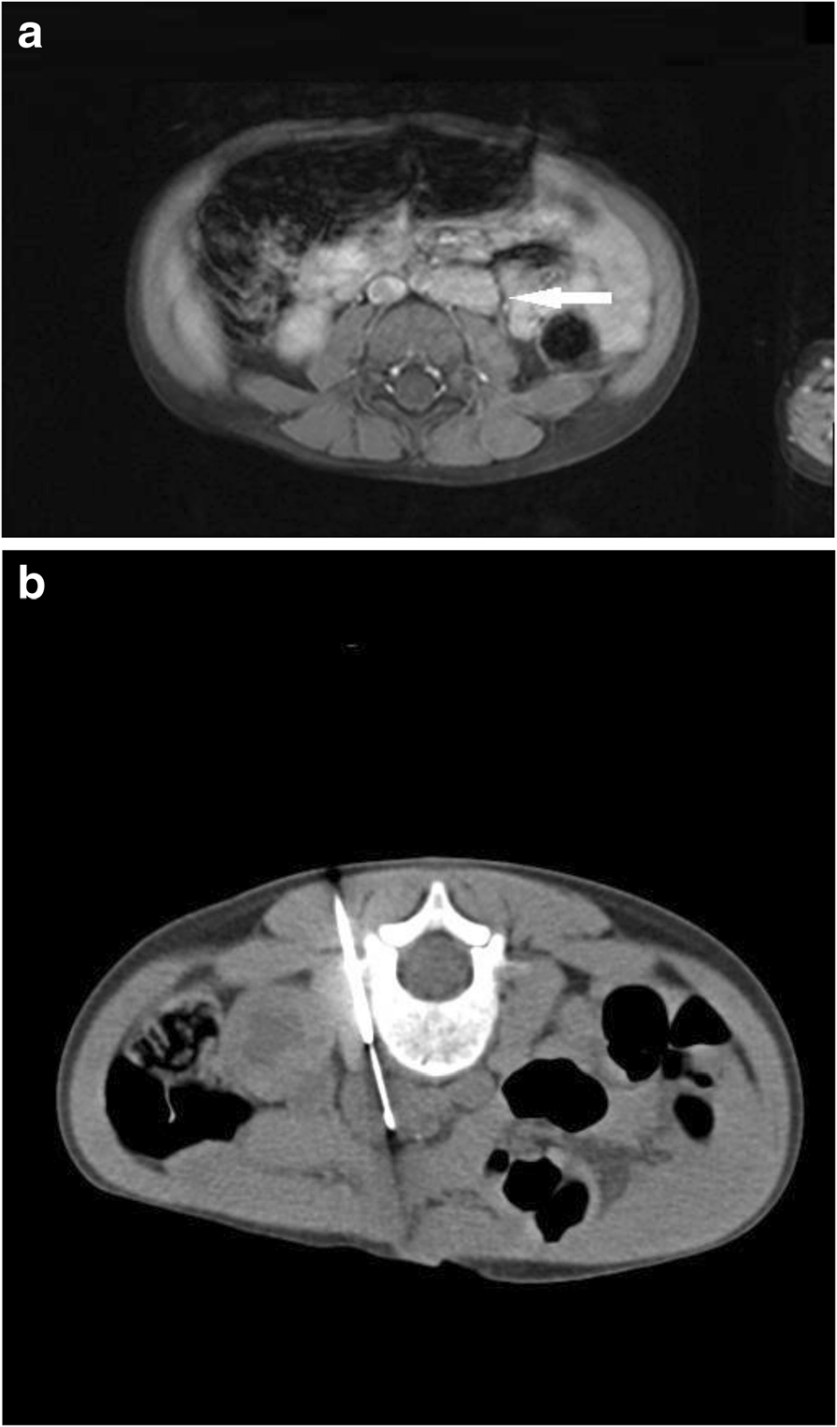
Four years old child with recurrent urinary tract infections. In MRI an unknown solid retroperitoneal masses on the left was documented. The size of the masses was 15 × 26 mm. Because of proximity the lesion to aorta and because of better controllability the intervention was performed with CT guidance. No complications were documented. The pathological report revealed a ganglioneuroma. a. Enhanced transverse T1-weighted MR image with fat saturation shows a suspect lesion paraaortal on the left (*arrows*). b. CT-Image documented a location of biopsy needle in the lesion
